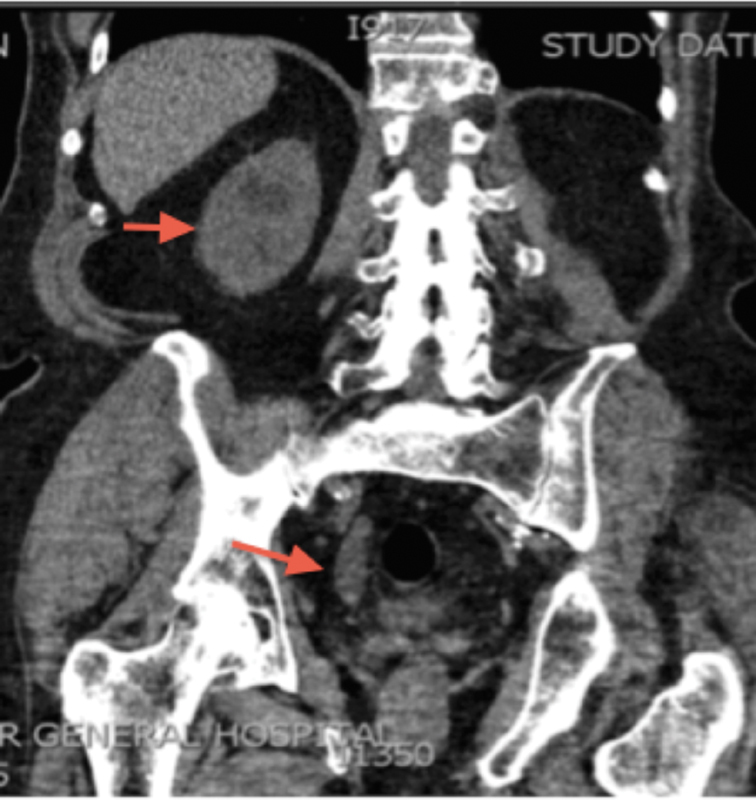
Coronal section: computed tomography of kidneys, ureters, and bladder showing the hydronephrotic kidney and dilated lower end of the ureter with narrowing at the vesicoureteric junction (arrows).
